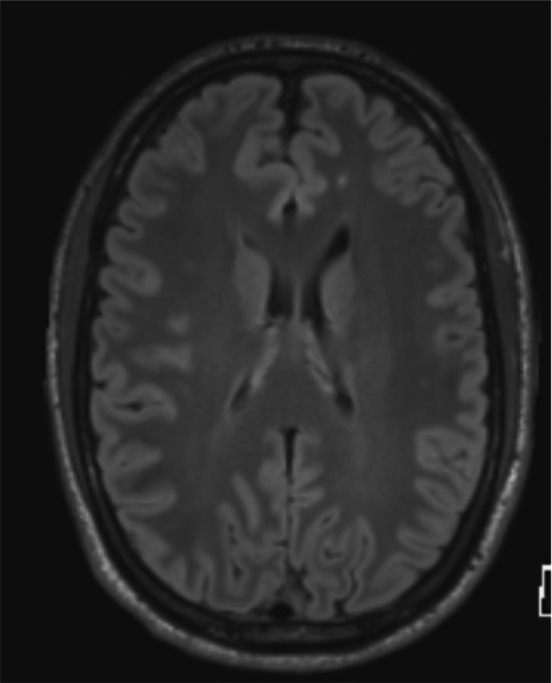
Example of white matter changes. T2 FLAIR image showing multifocal white matter changes.
